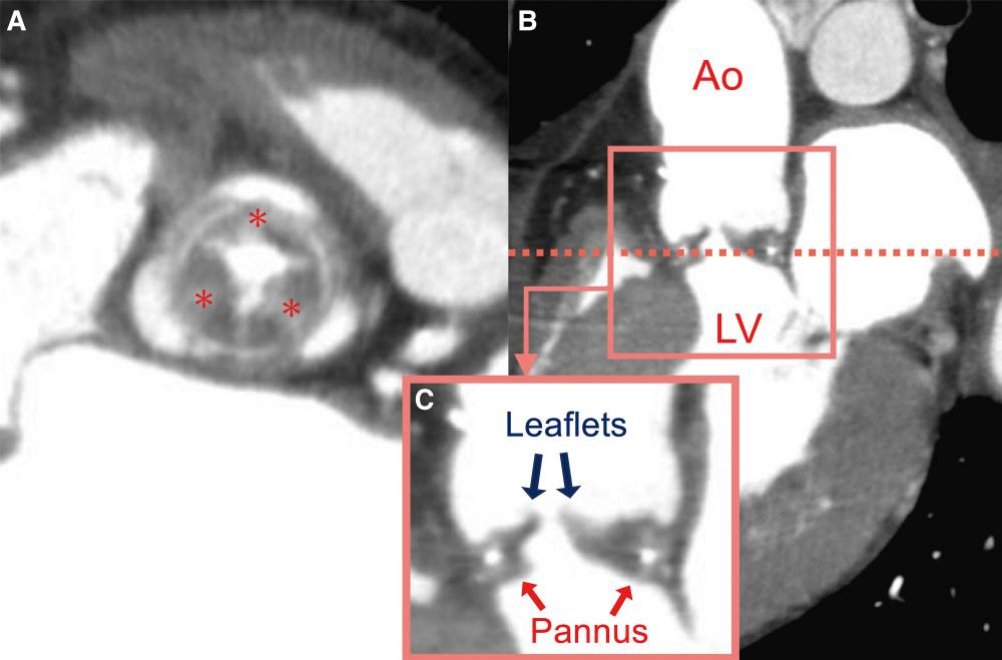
(A)* Short axis view of the bioprosthetic valve in contrast-enhanced computed tomography. This image is a cross-section at the level of the dotted line in *(B*). Asterisks: pannus beneath all three valve leaflets. (*B*) Sagittal view of the prosthetic valve (Ao, aorta; LV, left ventricle). (*C*) Enlarged view of the area enclosed by the square in *(B)*.
